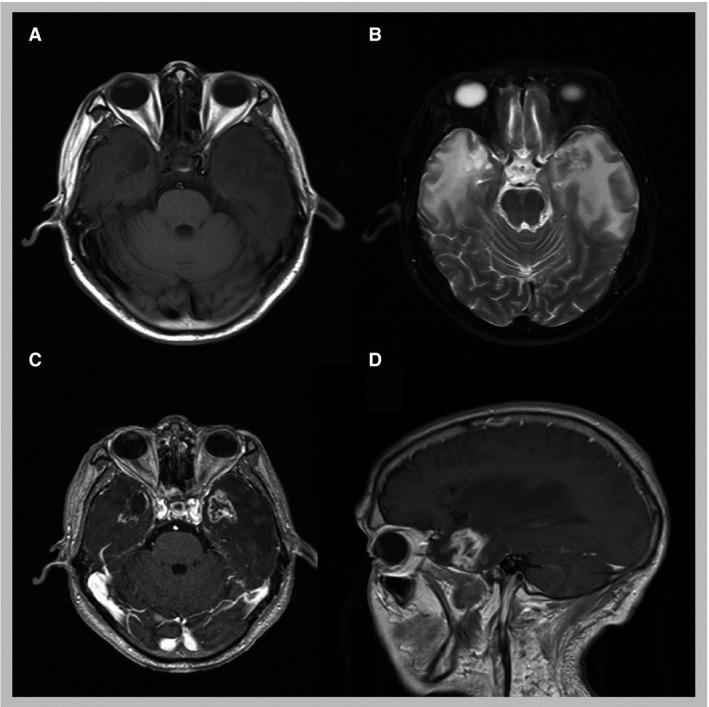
Typical MRI images for RITLI during follow up. RITLI, radiation‐induced temporal lobe injury. (A) Both temporal lobes showed decreased signal intensity on T1‐weighted image, especially for right temporal lobe; (B) Both temporal lobes showed increased signal intensity on T2‐weighted image; (C) Both temporal lobes showed contrast‐enhanced irregular lesions on T1‐weighted image in the axial plane; (D) Both temporal lobes showed contrast‐enhanced irregular lesions on T1‐weighted image in the sagittal plane.
